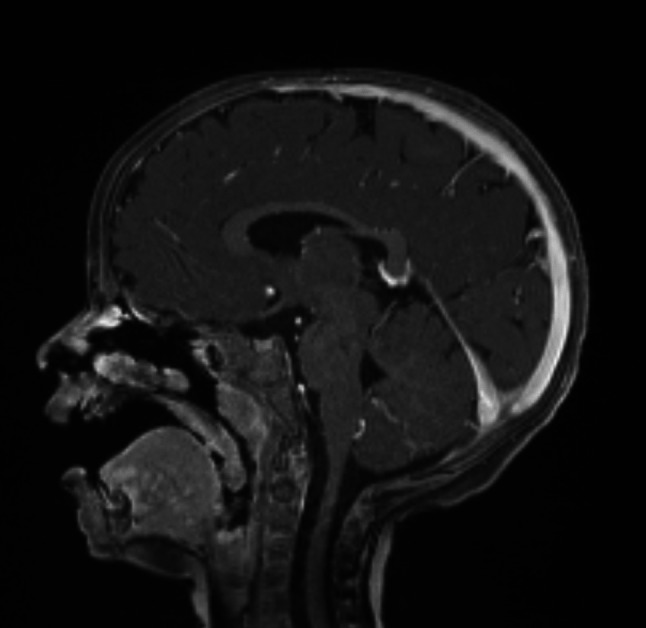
T2 weighted MRI scan in sagittal plane ten months after surgery
